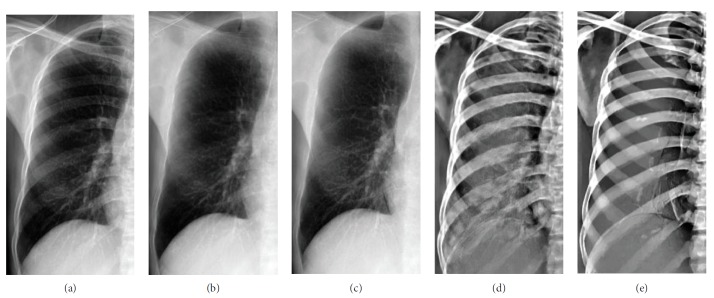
Decomposition results of a chest radiograph without motion artifacts: (a) the right lung field of a chest radiograph; (b) the decomposed soft tissue image; (c) the actual DES soft tissue image; (d) the decomposed bone image; (e) the actual DES bone image.
